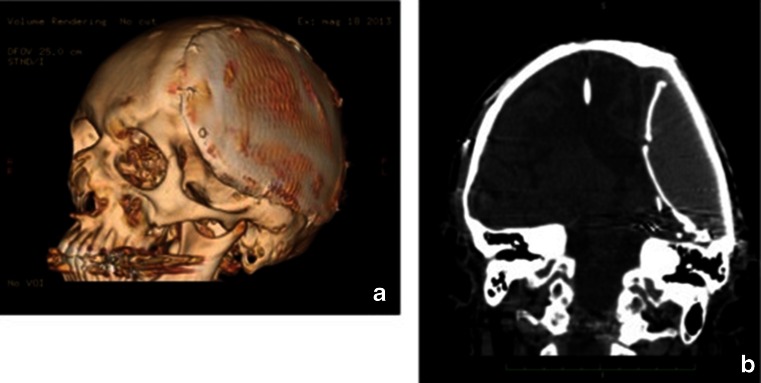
3D VR (a) and coronal CT reformat (b). Both views demonstrate nearly seamless contour between the tantalium plaque and the native cranial bone with the intended “flattened” cranial contour. The calcified Lyodura is well seen on the coronal view (b) as well as the uniform soft tissue density within the implant
